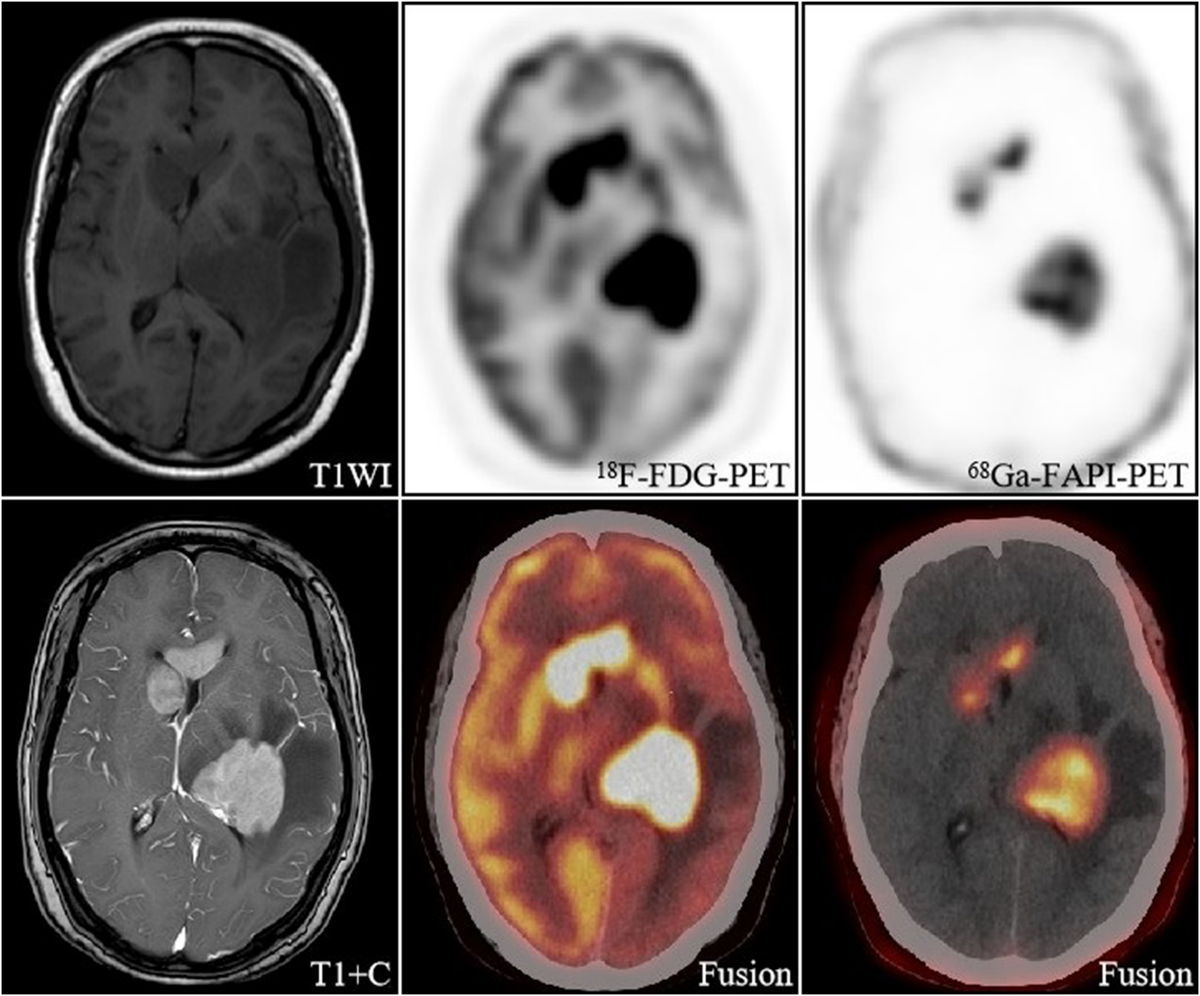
A 57-year-old woman, with a final diagnosis of intracranial diffuse large B-cell lymphoma. The intracranial lesions had a high uptake of [^18^F]FDG (SUVmax 8.5-32.1) and a much lesser degree and extent of uptake of [^68^Ga]FAPI than [^18^F]FDG, but still had a high TBR (67.5-78) due to a low background.
